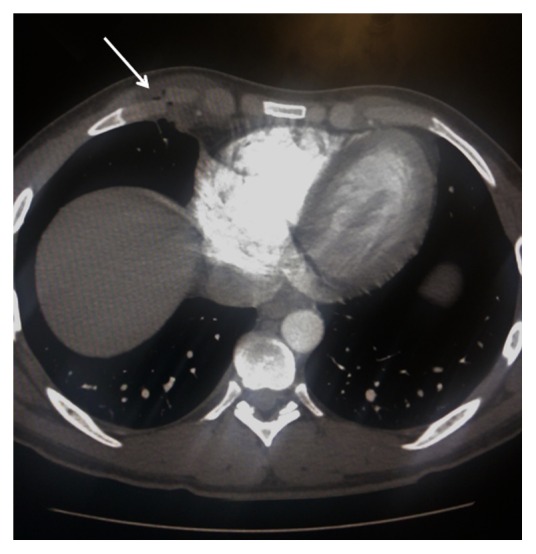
Axial contrast-enhanced CT 10 days after injury. Note the improvement in lung herniation (arrow).
